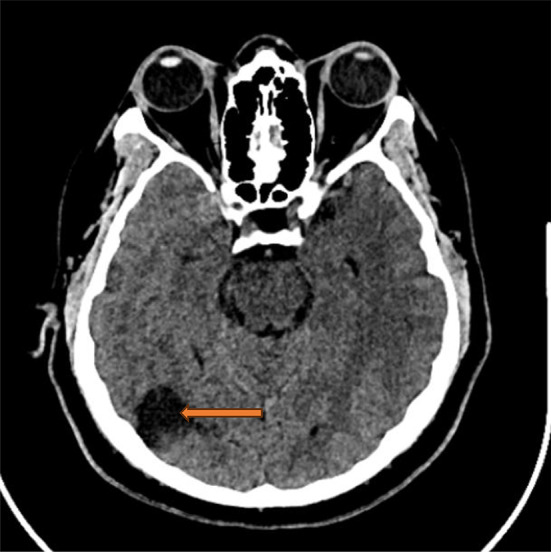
Follow-up non-contrast axial brain CT scan (4 months post-PRES). The image demonstrates chronic sequelae in the left occipital region (brown arrow), showing low attenuation consistent with encephalomalacia resulting from prior posterior reversible encephalopathy syndrome. The previously seen intraventricular hemorrhage has resolved, with the ventricular system now filled with cerebrospinal fluid. In this case, PRES was not reversible, contrary to its traditional characterization, highlighting that the syndrome is not invariably reversible
